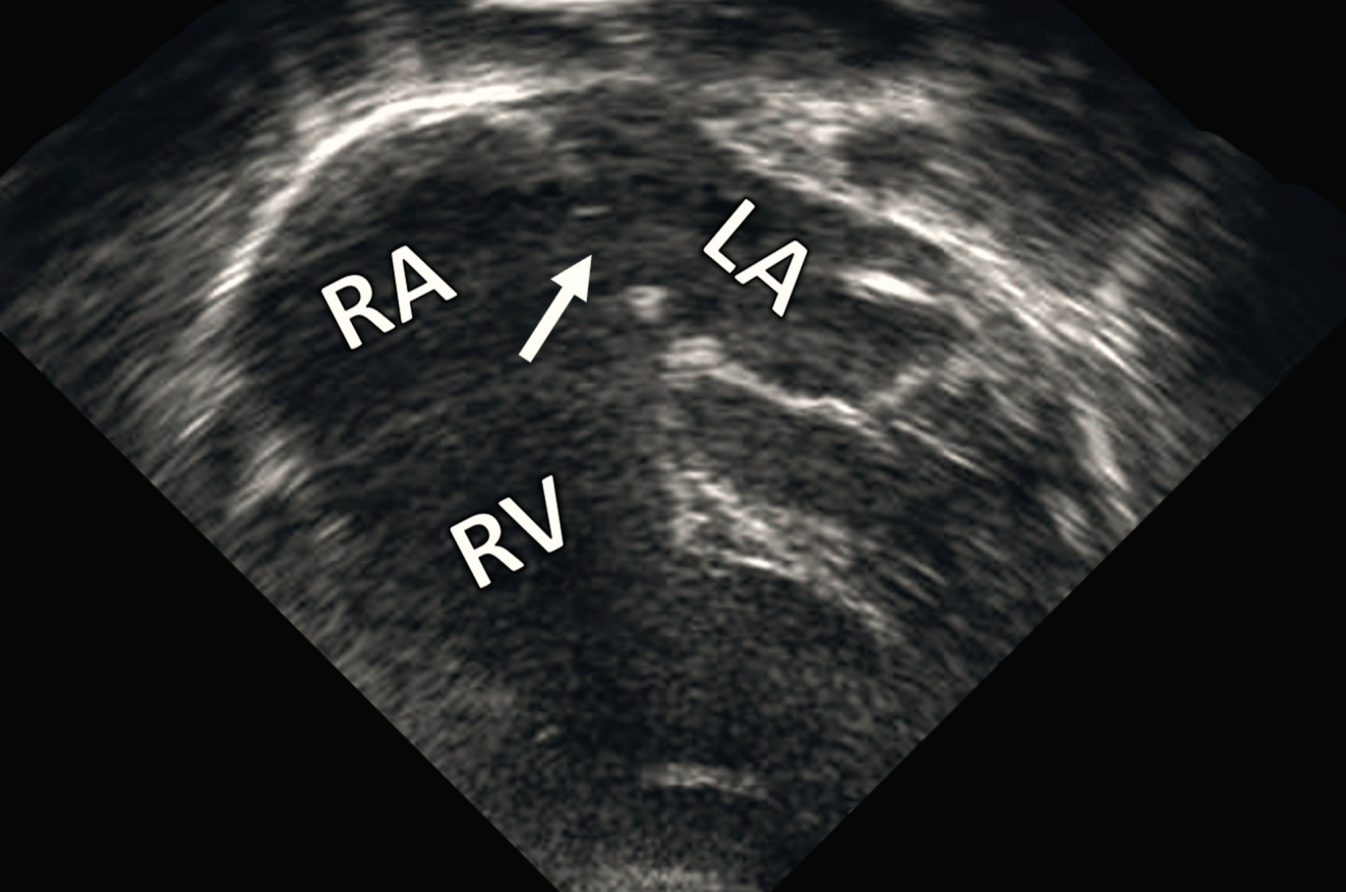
Echocardiography Echocardiographic image showing a moderate atrial septal defect (arrow). RA: right atrium; LA: left atrium; RV: right ventricle.
